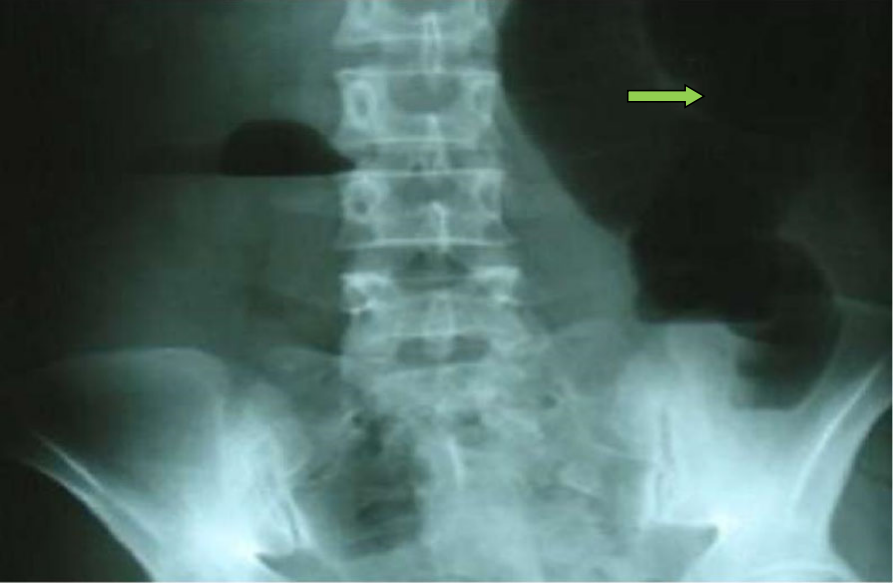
Abdominal X-ray showing (arrow) a dilated colon with a “U-shaped” loop in the left upper abdomen
